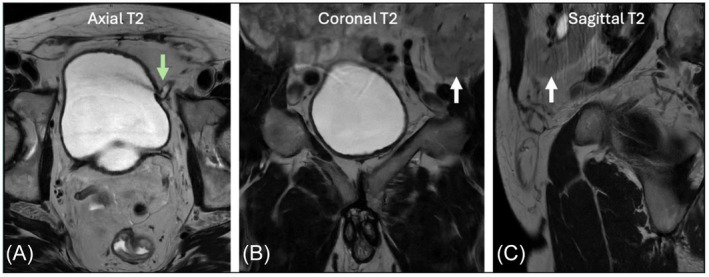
Title: prostate MRI demonstration of transplant kidney and ureter in the left iliac fossa. Caption: prostate MRI images of the same patient, showing a) the transplant ureter entering the left bladder wall at the site of implantation (green arrow), b) and c) the transplant kidney in the left hemi‐pelvis lateral to the bladder (white arrows).
